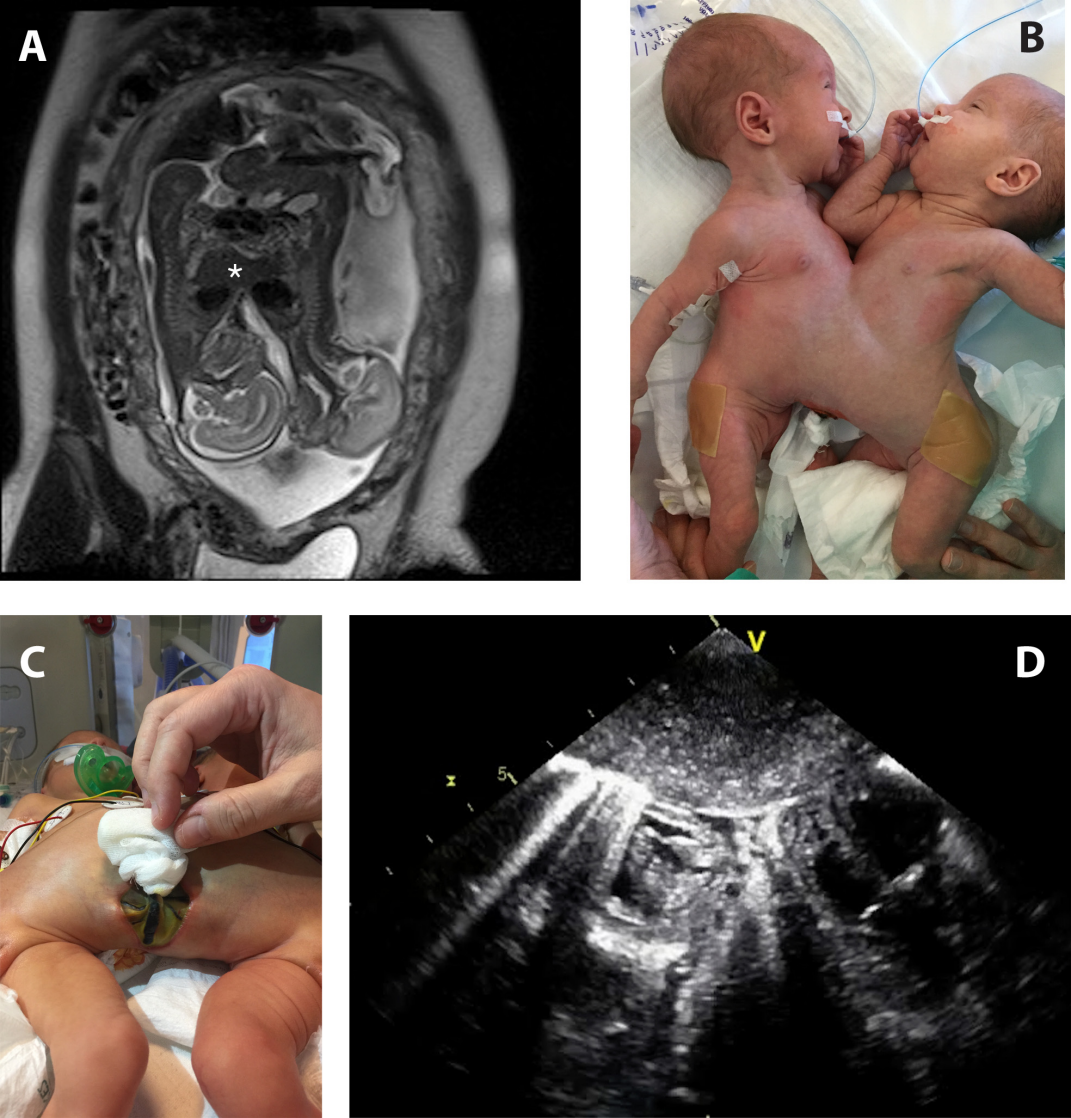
(A) A fetal T2 coronal magnetic resonance image (MRI) of conjoined omphalopagus twins at 21 weeks of gestation. A single liver is shared between both fetuses (*). Each fetus has a separate heart, stomach, kidneys, and urinary bladder. (B) and (C) Photographs of the twins. (D) A two-dimensional echocardiography image before separation showing separate hearts and pericardium. The heart apexes pointing toward each other (heart of the twin B is rotated to the right with the apex pointing toward the apex of twin A, which is in the normal left position). The distance between the apexes is 5-6 mm. The hearts were separated with the lower part of the sternum.
